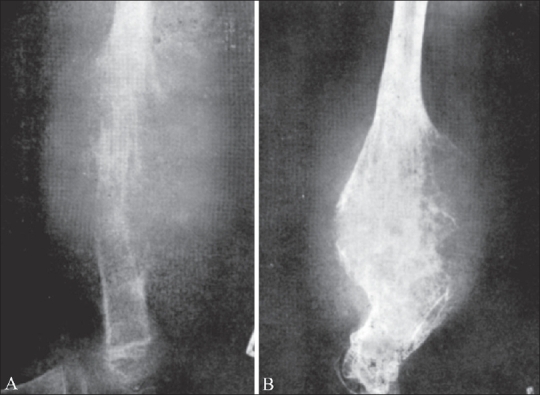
Ewing's sarcoma of the femur. Pre-irradiation radiograph (A) shows a large, destructive lesion, showing a bit of a “sun-ray” appearance. Post-irradiation radiography (B) shows bone condensation and healing
